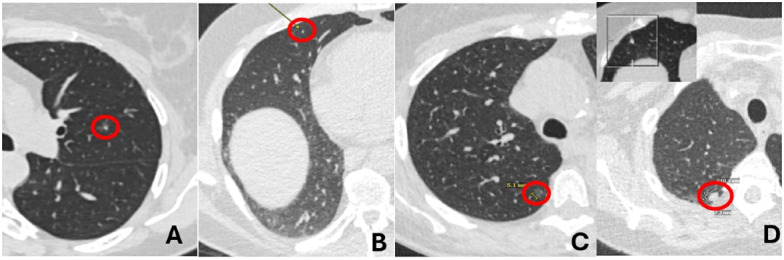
Transverse chest CT images demonstrating discrepancies between AI outputs and radiology reports for the following nodules: (A) A 4 mm left upper lobe nodule in a 50-year-old female patient was missed by the AI (complete discordance due to missed actionable nodule); (B) AI detected no nodules in a 57-year-old man with a 2 mm right middle lobe nodule in the radiology report (complete discordance due to missed actionable nodule); (C) AI measured the right upper lobe ground-glass nodule as 8 mm versus 5 mm in the radiology report (partial discordance: overestimation of non-actionable nodule); (D) AI reported an 8 mm right middle lobe nodule (inset) in a 71-year-old female patient, while the report described a 9 mm right upper lobe nodule as the actionable nodule (complete discordance from misdetection of actionable nodule). AI: artificial intelligence; CT: computed tomography.
